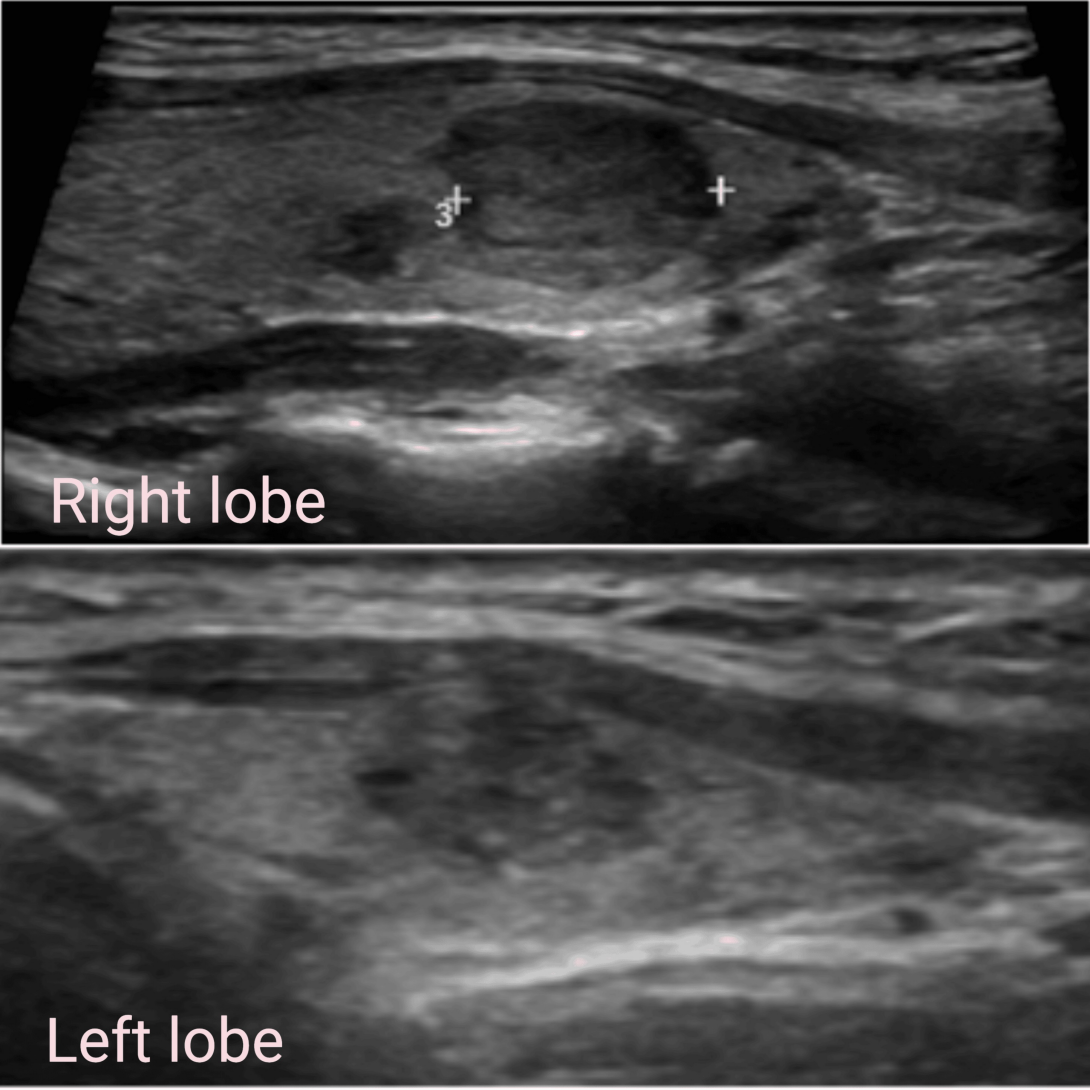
Ultrasound showing nodules in right and left lobe of thyroid
